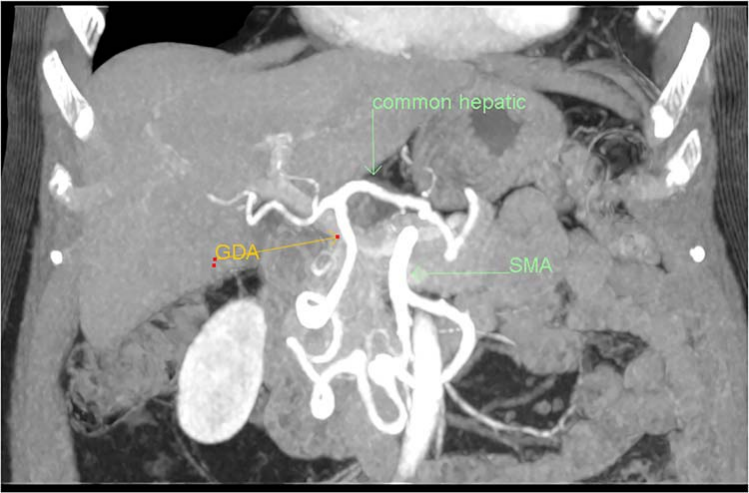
Multiphase CT pancreas, coronal view: the gastroduodenal artery, off the common hepatic, communicating with the first branch of the SMA.
